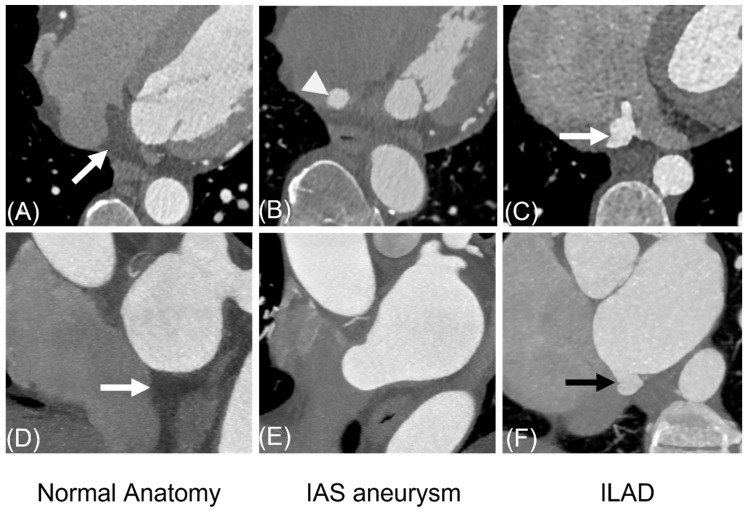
Comparison among normal anatomy, interatrial septal aneurysm (IASA), and ILAD on transverse (upper panel) and short-axial (lower panel) CT images. The wedge-like fatty space (white arrows in (A,D) remains intact in healthy individuals and those with IAS (B,E), where the aneurysmal area (arrowhead in (B)) is restricted to the right atrium. In contrast, ILAD (white and black arrows) (C,F) fills this fatty space. Abbreviations: IASA, interatrial septal aneurysm; ILAD, inferior left atrial diverticulum.
